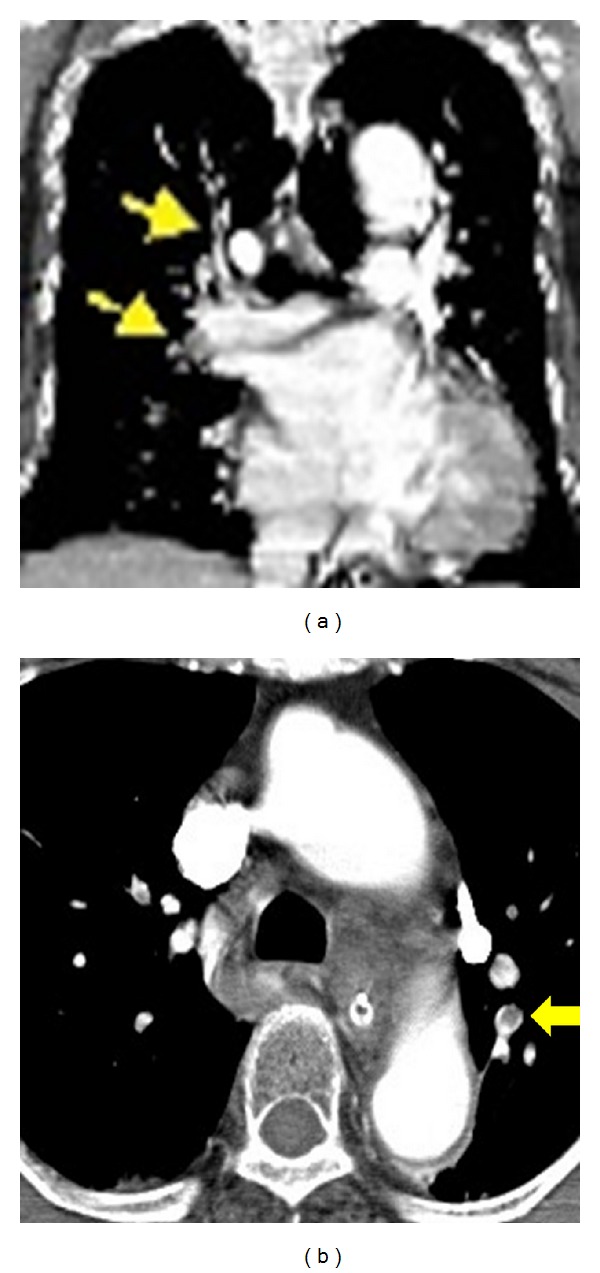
Contrast-enhanced CT of the lung. The coronal reconstructed image (a) revealed thrombi in the superior and inferior lobe branches of the right pulmonary artery (yellow arrows). The transverse image (b) showed a thrombus in the superior lobe branch of the left pulmonary artery (yellow arrow).
